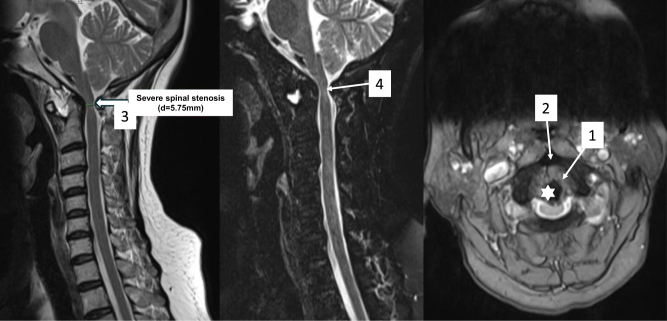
Cervical MRI revealed severe spinal stenosis at the level of C1–C2 (an anteroposterior diameter of 5.75 mm. On sagittal T2-STIR of MRI, the hyperintense signal within the spinal cord at the current level indicates spinal cord edema (arrow 4). On axial plane, the C2 odontoid process shifting to the center of the spinal canal directly compression to the spinal cord causing commensurate edema (star: C2 odontoid process; arrow 1: the interval between C1 anterior arch and C2 odontoid; arrow 2: C1 anterior arch; arrow 3: anteroposterior diameter of spinal cord).
